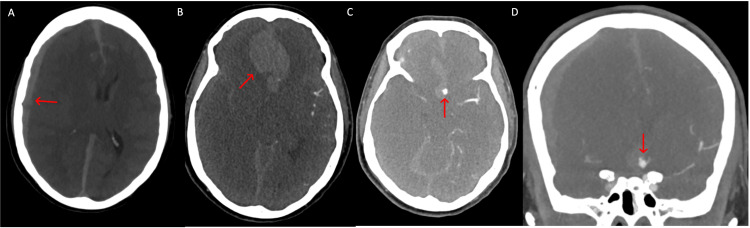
A: Preoperative axial CT demonstrating interhemispheric and convexity subdural hematoma >8 mm (red arrow) with mass effect and bilateral acute subarachnoid hemorrhage, B: Preoperative axial CTA demonstrating large bifrontal intraparenchymal hemorrhage (red arrow), C: Preoperative axial CTA demonstrating 4 mm left sided anterior communicating artery aneurysm (red arrow) with surrounding intraparenchymal hemorrhage, tentorial subdural hemorrhage, and bilateral subarachnoid hemorrhage, D: Preoperative coronal CTA with left convexity subdural hematoma, anterior communicating artery aneurysm (red arrow), and surrounding intraparenchymal hemorrhage
